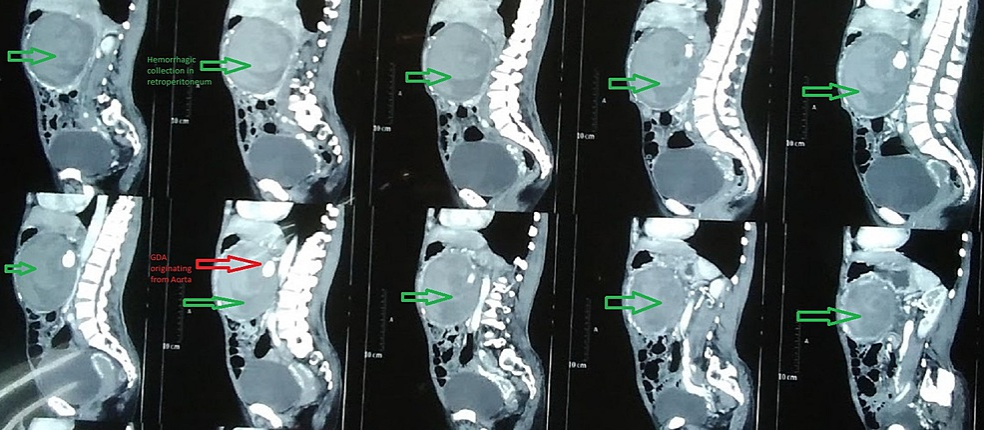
CT: Sagittal sections showing anomalous origin of the GDA from the aorta. CT: computed tomography; GDA: gastroduodenal artery
